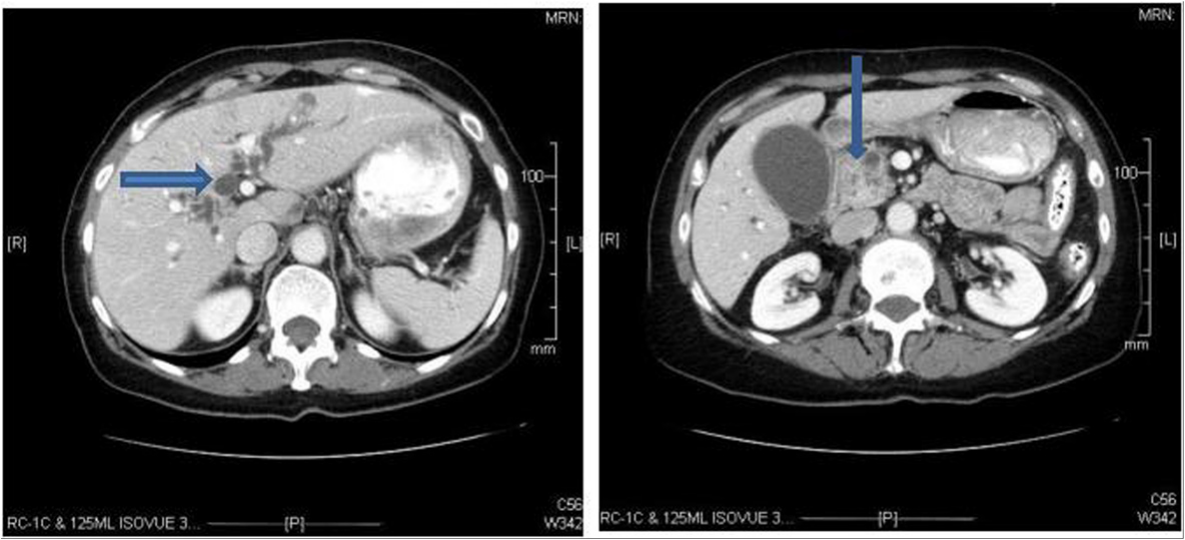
Representative cuts of the patient’s initial CT scan demonstrating intrahepatic biliary ductal dilatation (a) and a heterogeneous mass within the head of the pancreas (b)
